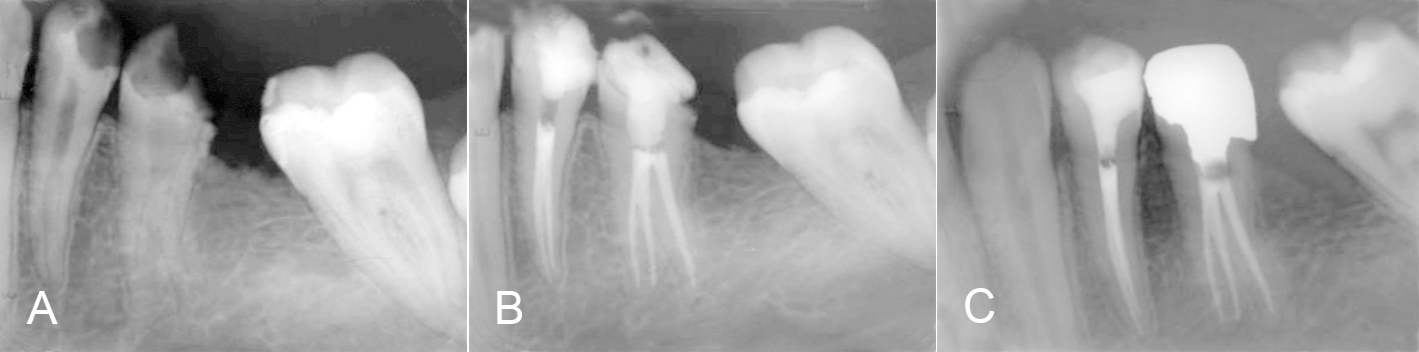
A: Three-canal second premolar. B: Immediate post operative radiograph showing three canals with three portals of exit. C: One-year recall.
